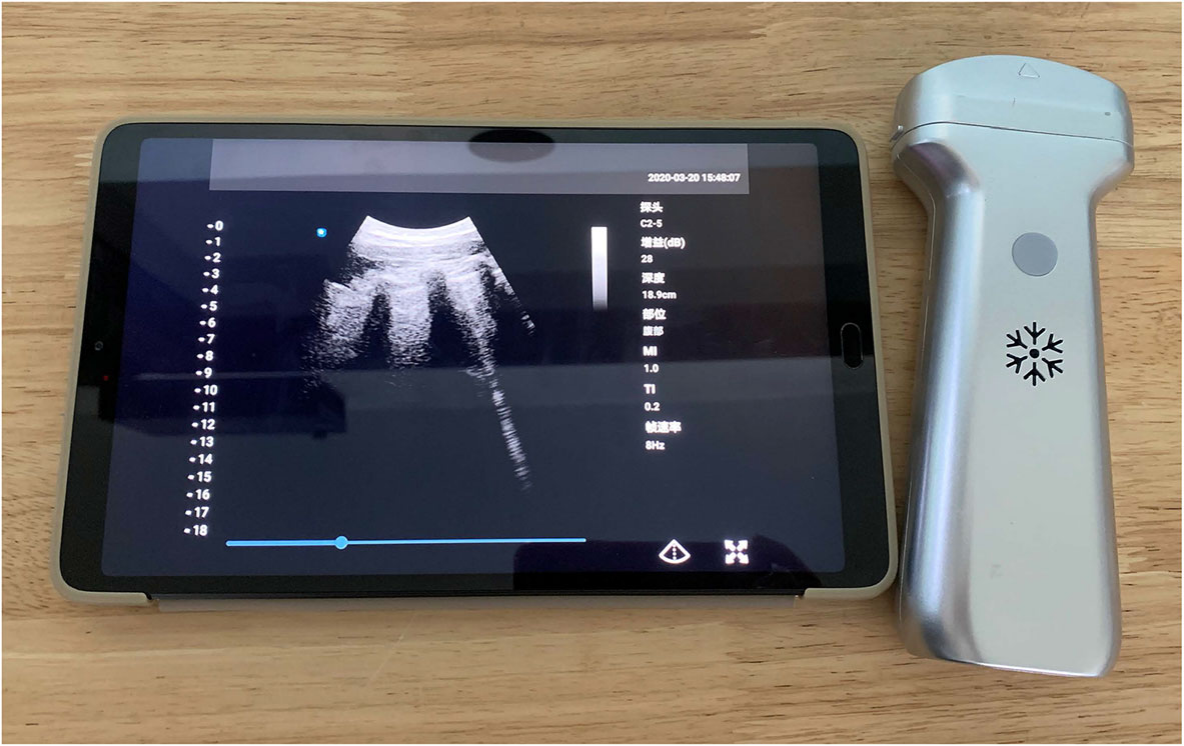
The handheld ultrasound device
